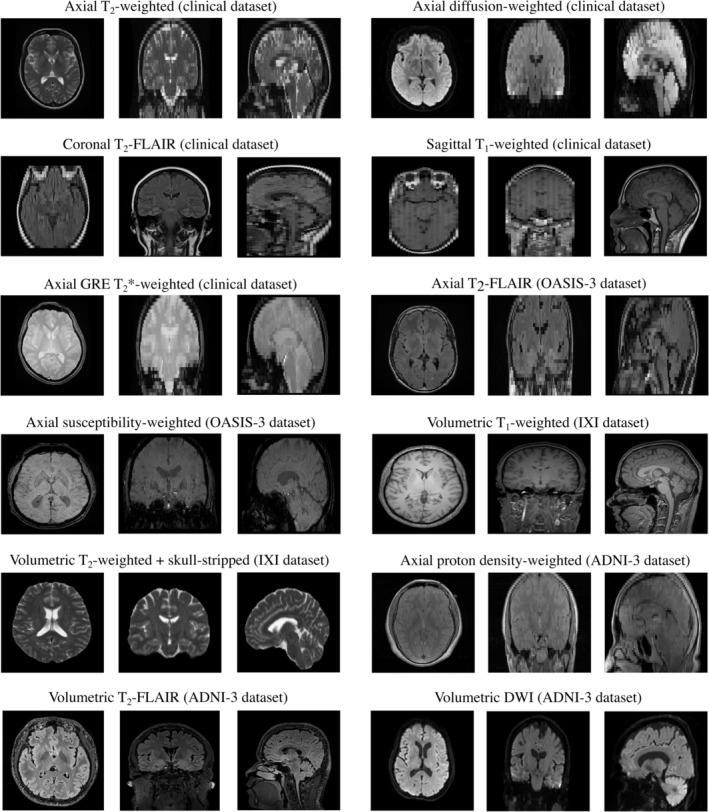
Overview of the different types of head MRI scans used for brain age modelling in this study. A transfer learning experiment using skull‐stripped data is described in Section 2.2.
